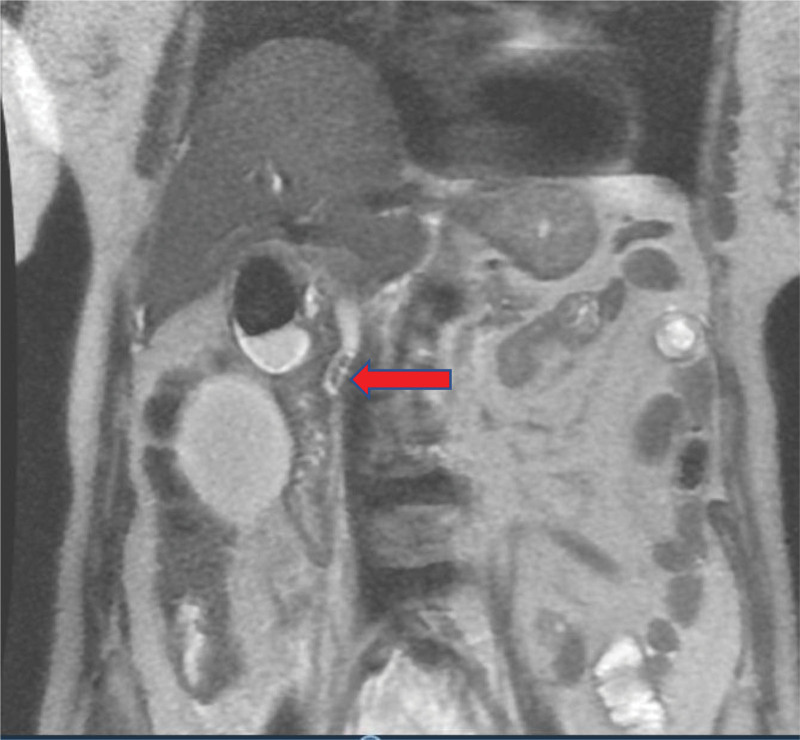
Coronal magnetic resonance imaging (MRI) showing a dilated (12 mm) common bile duct with a multiple filling defects in the proximal common bile duct (red arrow) indicating lodged stones.
